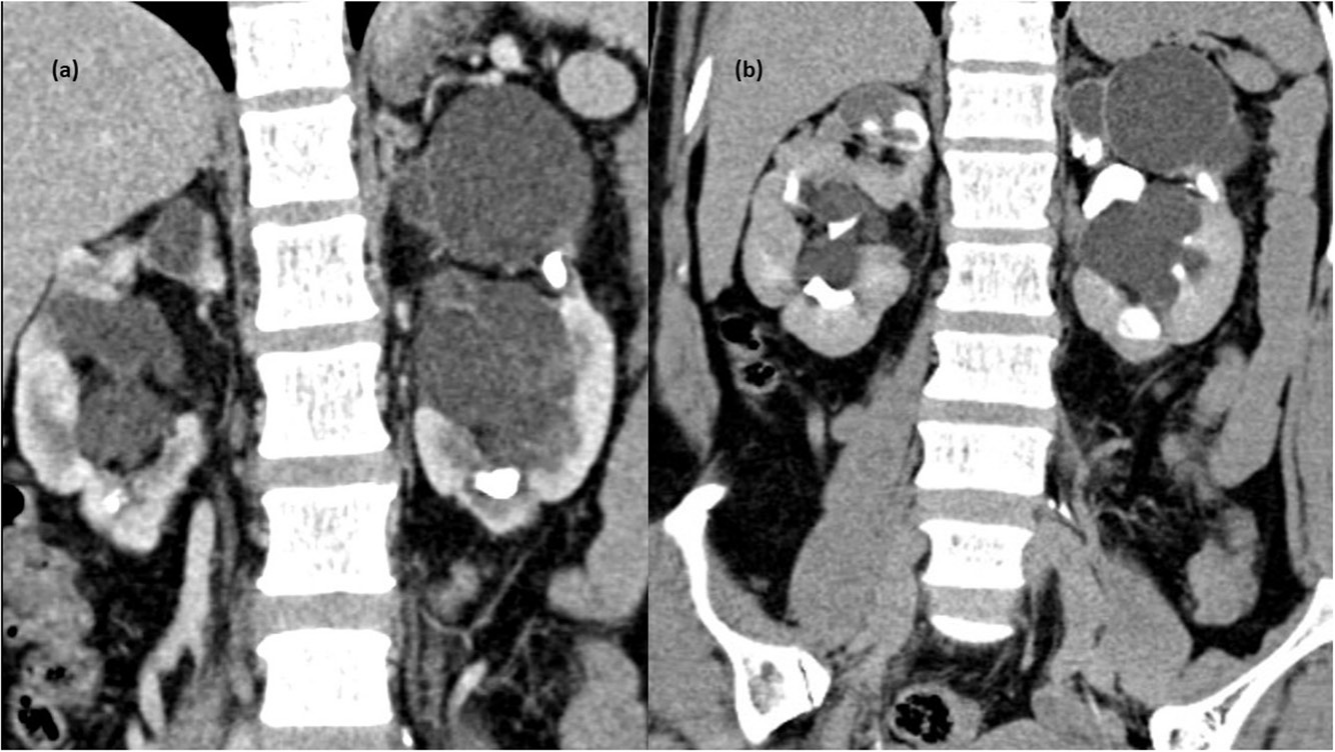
CT scan venous phase in coronal plane showing nonenhancing collection in peripelvic region of both kidneys (d) MPR reformation of coronal plane of delayed phase of CT scan abdomen showing normal excretion of contrast with no evidence of leakage in PC system. Bilateral peripelvic lymphangiectasia. Associated cystic lesions are also seen.
